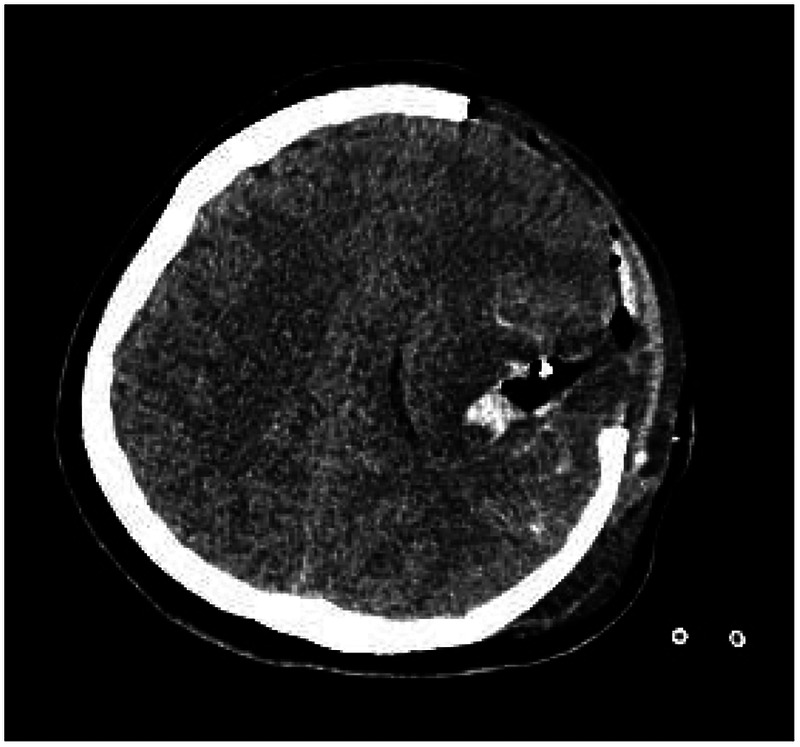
Postoperative CT shows a left frontotemporal craniectomy with intracranial air, surgical clips, and drainage catheters *in situ*.
